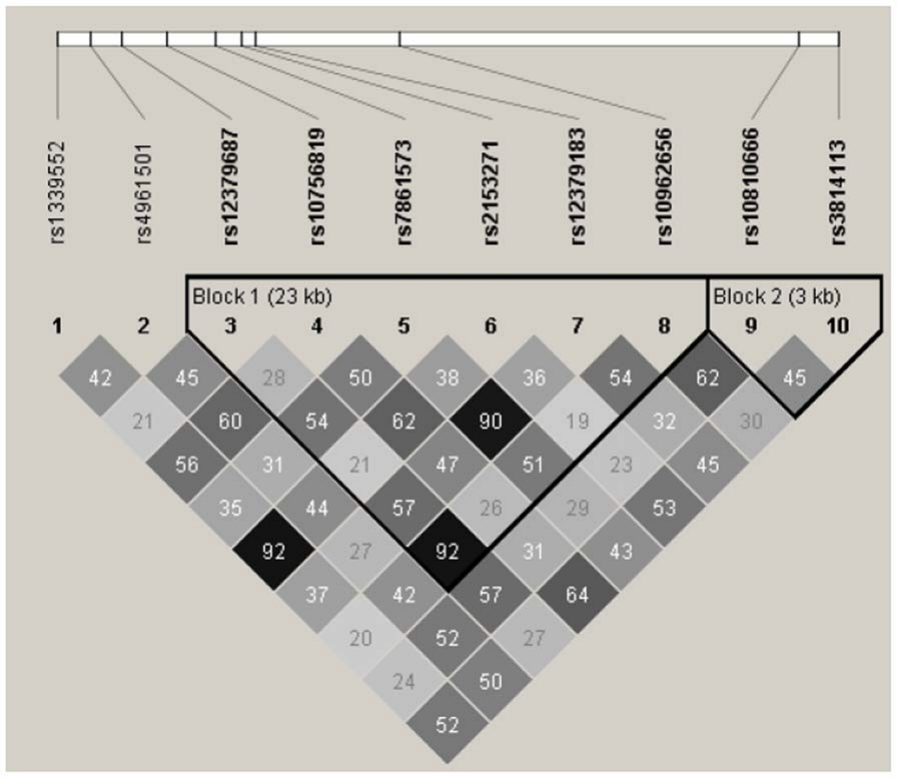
LD-plot of 10 SNPs on 9p22 from individuals included in the analysis. The LD-plot was generated with Haploview based on r^2^ of the 10 SNPs on 9p22 in 992 Caucasian women with genotyping information and transvaginal ultrasound results available.
